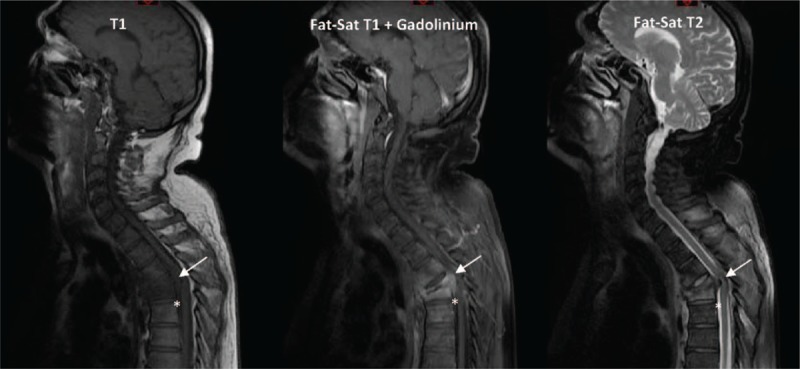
Magnetic resonance imaging (MRI) in pyogenic vertebral osteomyelitis. Fat-Sat: T1 or T2 sequence with saturation of fat signal. Vertebral osteomyelitis show intervertebral disc and adjacent vertebrae hyposignal on T1 sequence, contrast enhancement in T1 sequence with Gadolinium injection. Nucleus of intervertebral disc and adjacent vertebrae appear in hypersignal on T2 sequence. T4-T5 pyogenic vertebral osteomyelitis with disc disappearance, vertebral body destruction, kyphotic angulation, epidural inflammation (^∗^), and spinal cord compression by an anterior epidural abscess (arrow).
